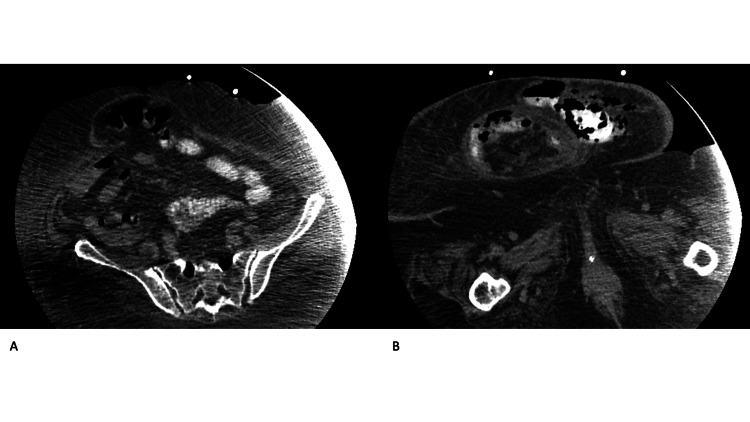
Computed tomography Α. Abdominal computed tomography showing a large periomphalic hernia with intestinal components, embedded in the adipose tissue of the abdominal wall. B. Presence of a second large abdominal wall hernia in the lower abdomen. There are signs of intestinal perforation in the abdominal wall (air bubbles in the adipose tissue), while a part of the intestine is in close proximity to the skin, indicating the presence of an enterocutaneous fistula.
